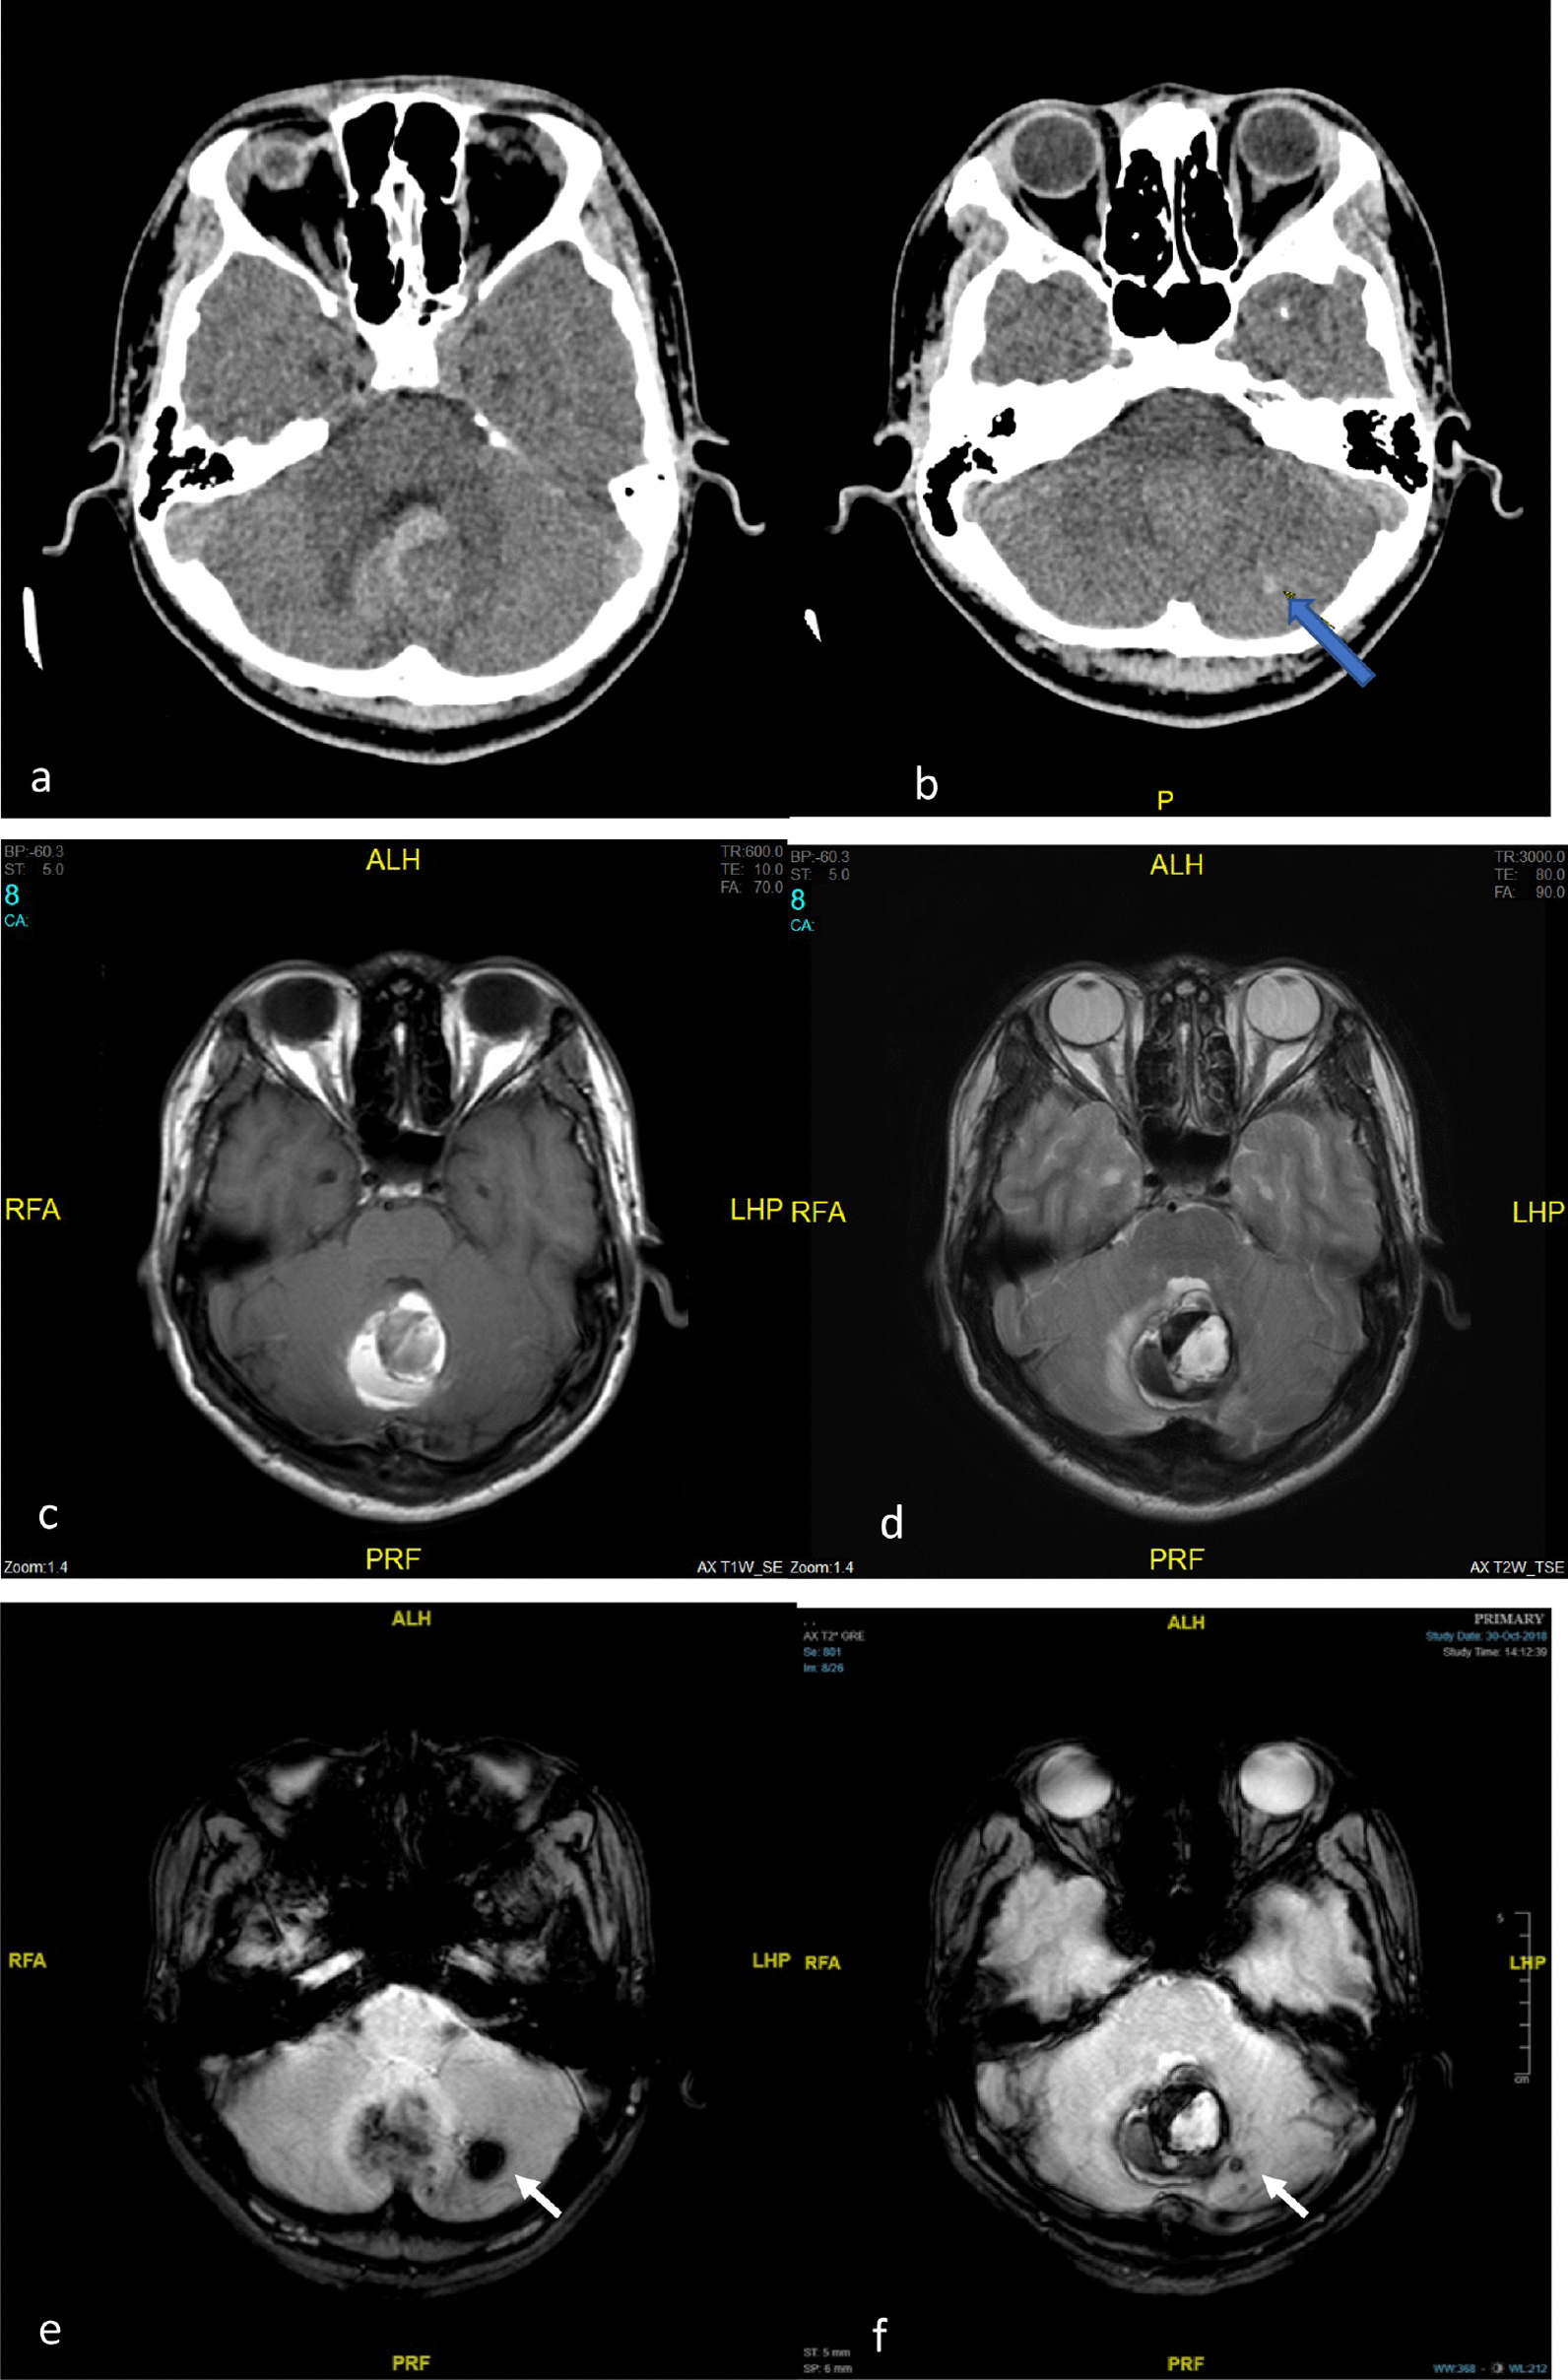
Non-contrast computed tomography brain (a) demonstrating cerebellar vermis hemorrhage. Contrasted computed tomography brain (b) showing enhancing area at left cerebellum, adjacent to the hemorrhage. Axial T1-weighted magnetic resonance imaging (MRI) (c), axial T2-weighted magnetic resonance imaging (d), and axial gradient echo sequence (e, f) demonstrating different ages of hemorrhage at cerebellar vermis. Multiple well-defined rounded blooming artifacts of varying sizes are seen in the left cerebellum, adjacent to the hemorrhage, representing cavernomas (white arrow in e and f)
